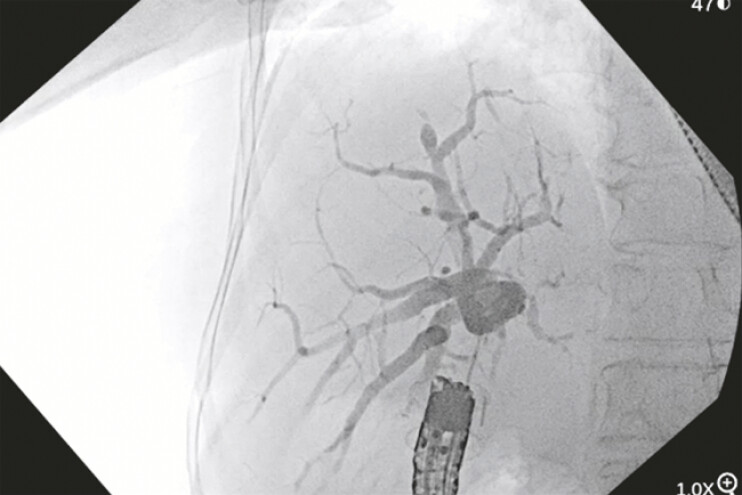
Endoscopic ultrasound (EUS)-guided cholangiogram showing successful puncture of the common hepatic duct.
